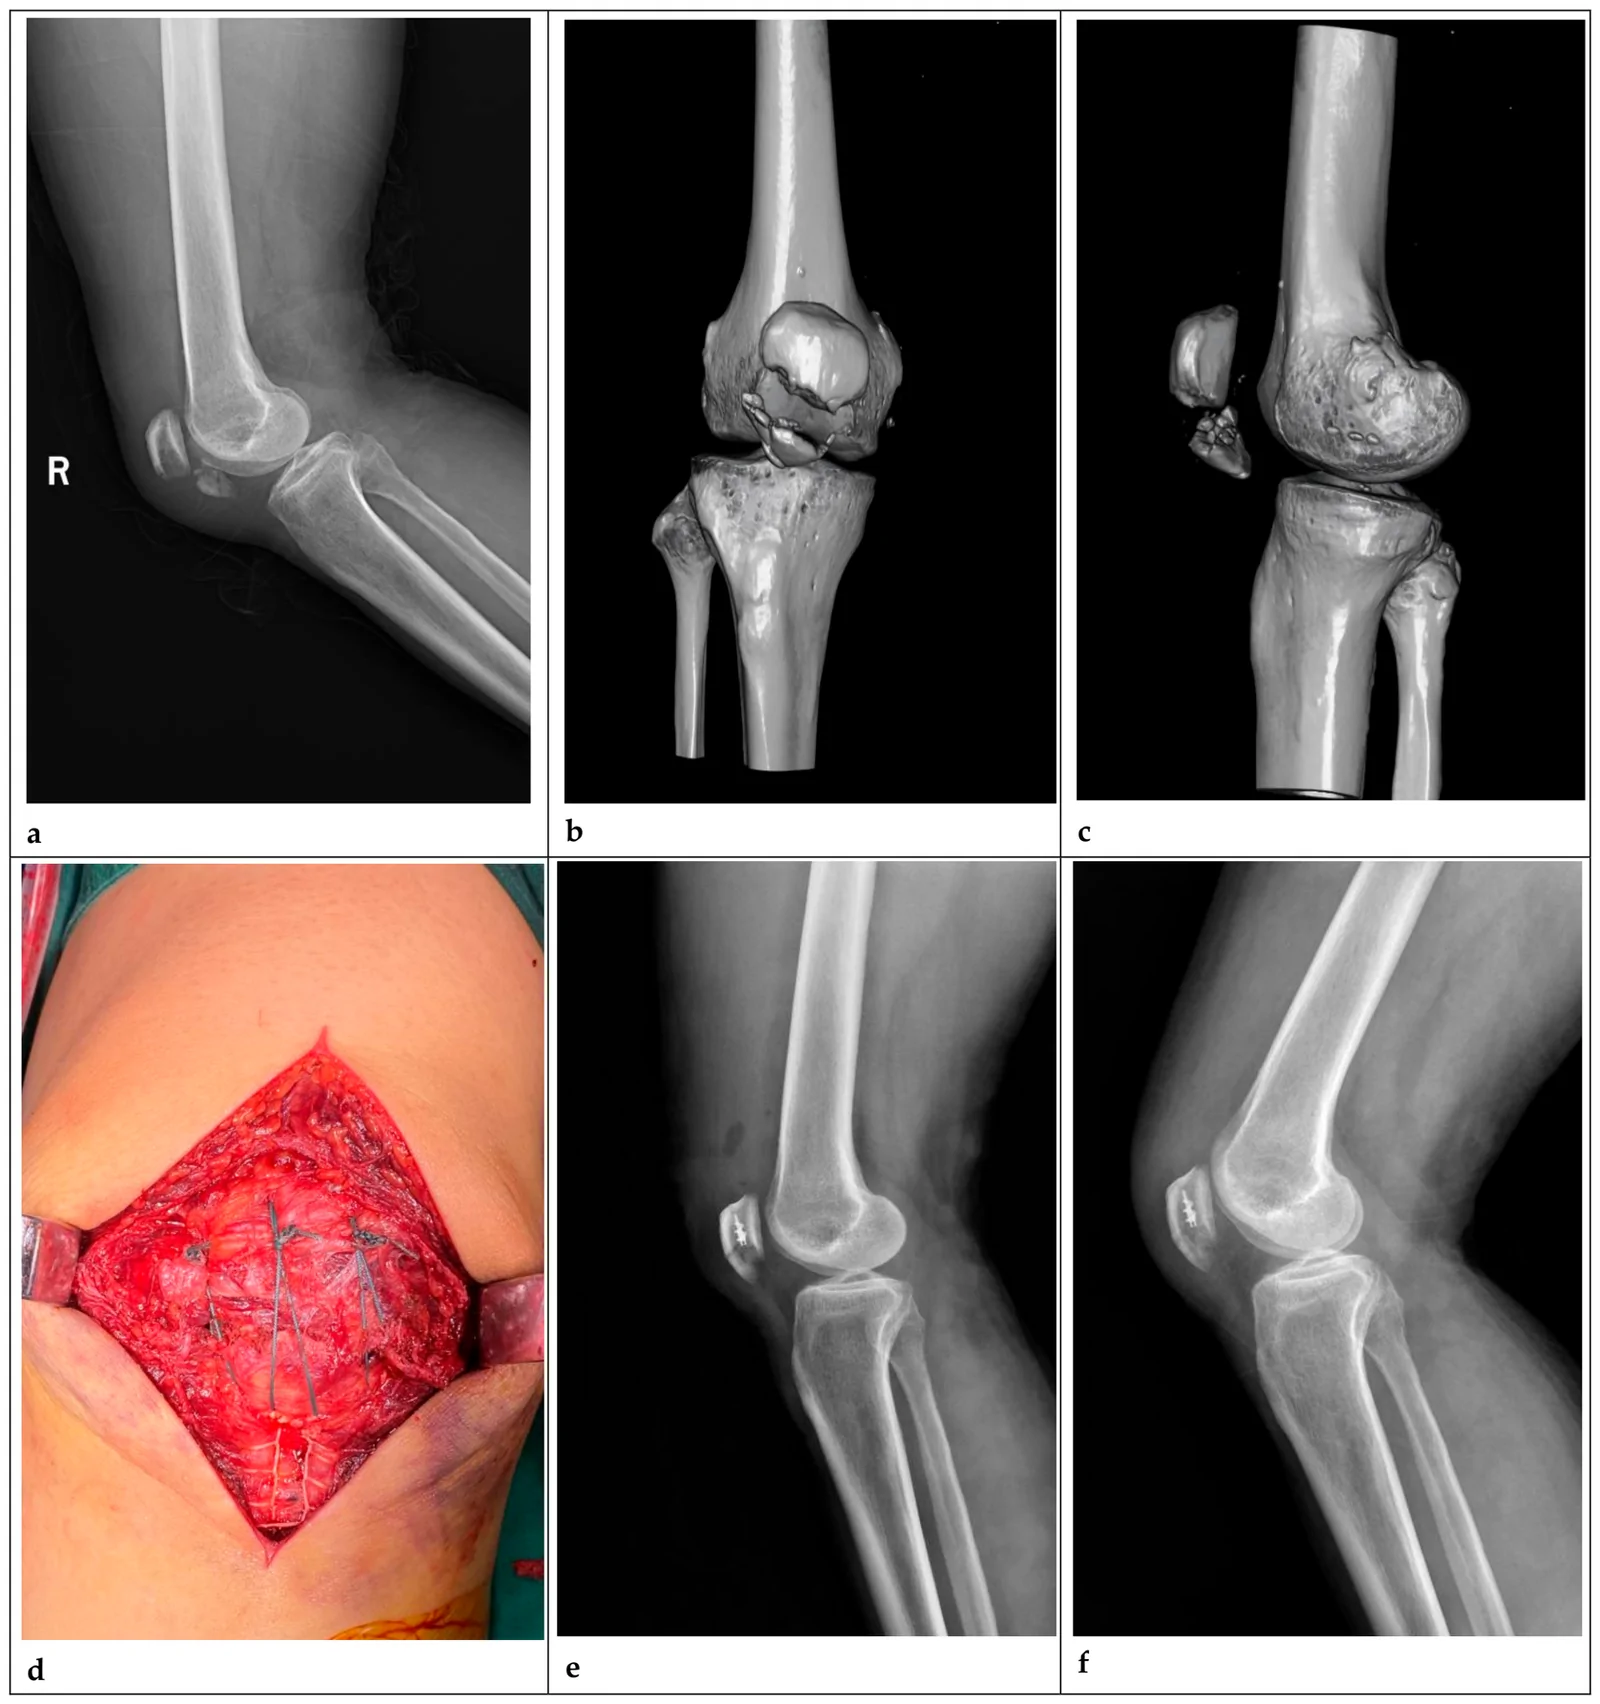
Preoperative and postoperative imaging of a right inferior patellar pole fracture. (a–c) Preoperative lateral radiographs and three-dimensional computed tomography (CT) images of the right knee show a displaced fracture of the inferior patellar pole. (d) Intraoperative view demonstrating reduction and fixation. (e) Immediate postoperative lateral radiograph reveals satisfactory fracture reduction and optimal anchor placement. (f) At the final follow-up, the lateral radiograph shows solid bone union.
